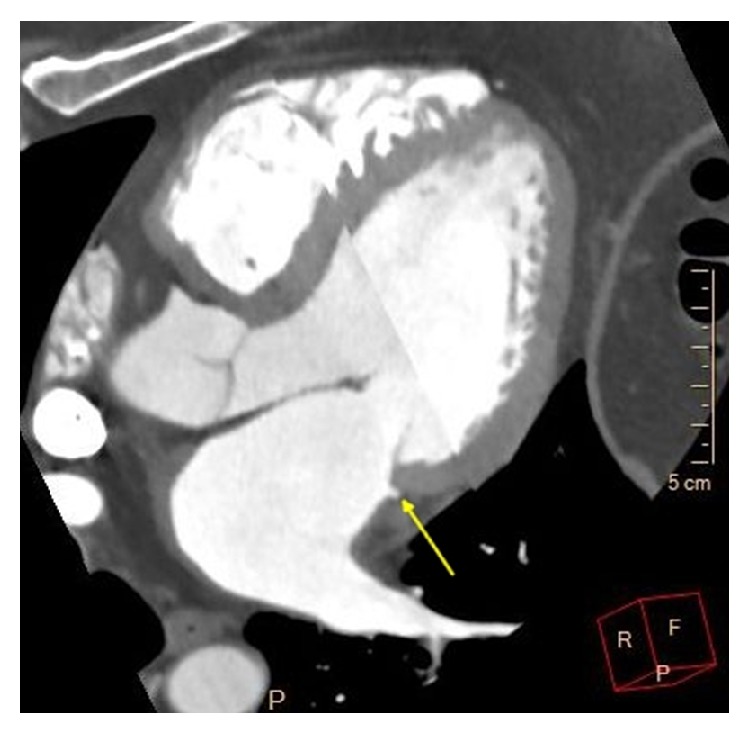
Contrast-enhanced CT image demonstrating a diverticulum (arrow) at the lateral part of the left atrium, close to the mitral valve.
